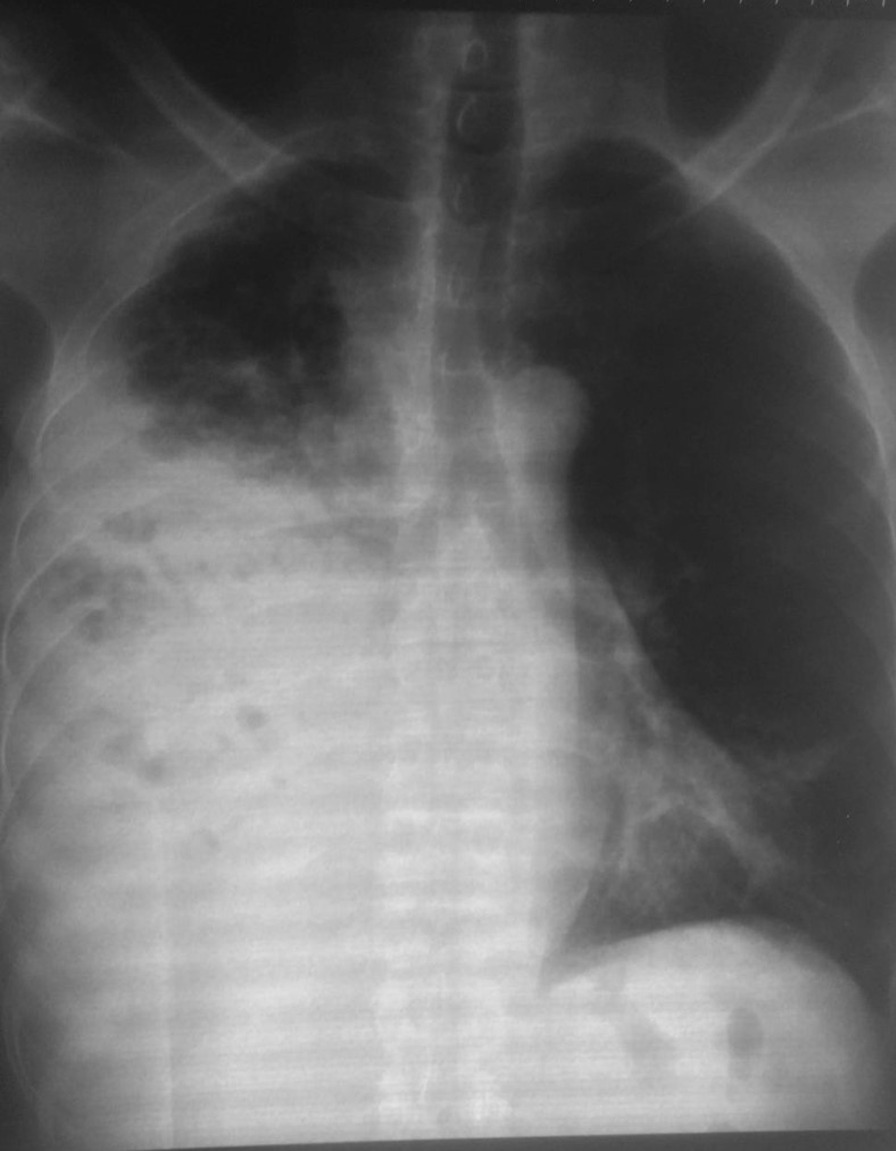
Chest x-ray (PA view) displaying consolidation on right middle to lower lung zones with areas showing bronchograms
